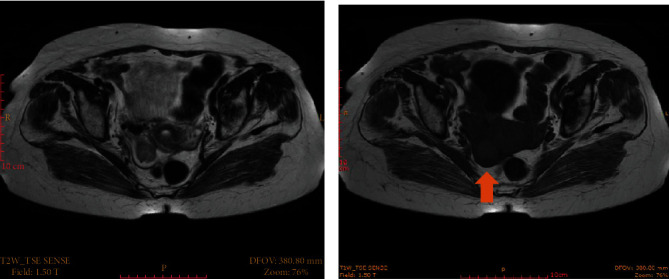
(a) Transaxial T2-weighted MRI shows a right adnexal mass (arrow) displaying thick wall with high signal intensity. (b) Loss of signal on T1-weighted image.
